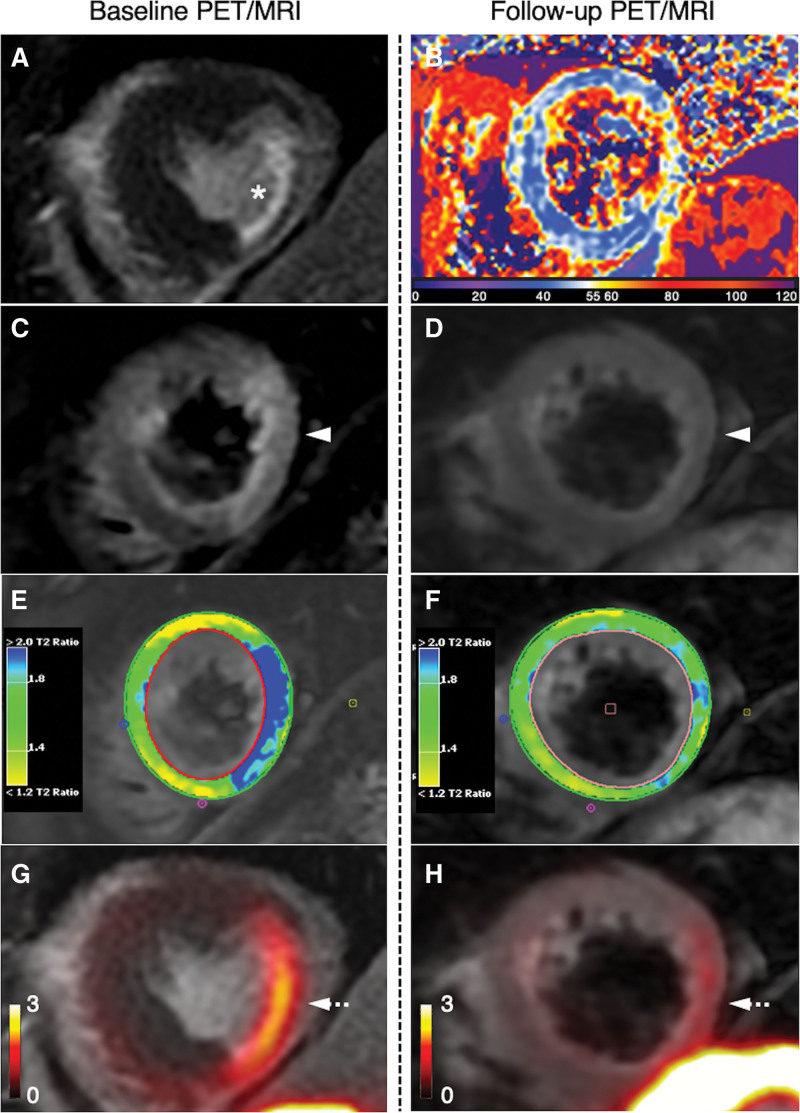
Comparison of baseline and 3-month follow-up ^68^Ga-DOTATATE positron emission tomography (PET)/magnetic resonance imaging (MRI). Baseline PET/MRI: (A) Late gadolinium enhancement (LGE) MRI showing sub-endocardial myocardial infarction (asterisk) in the mid inferolateral segment, with corresponding (C) high signal (arrowhead) on T2-weighted imaging; and (G) increased ^68^Ga-DOTATATE PET uptake (dashed arrow). E, Color-map demonstrates myocardium-to-muscle T2 ratio. Follow-up PET/MRI performed 102 days postinfarct: (B) T2-mapping; (D) resolution of edema on T2-weighted imaging; (F) myocardium-to-muscle T2 ratio; (H) residual infarct-related ^68^Ga-DOTATATE signal (dashed arrow). LGE imaging was not repeated at follow-up.
